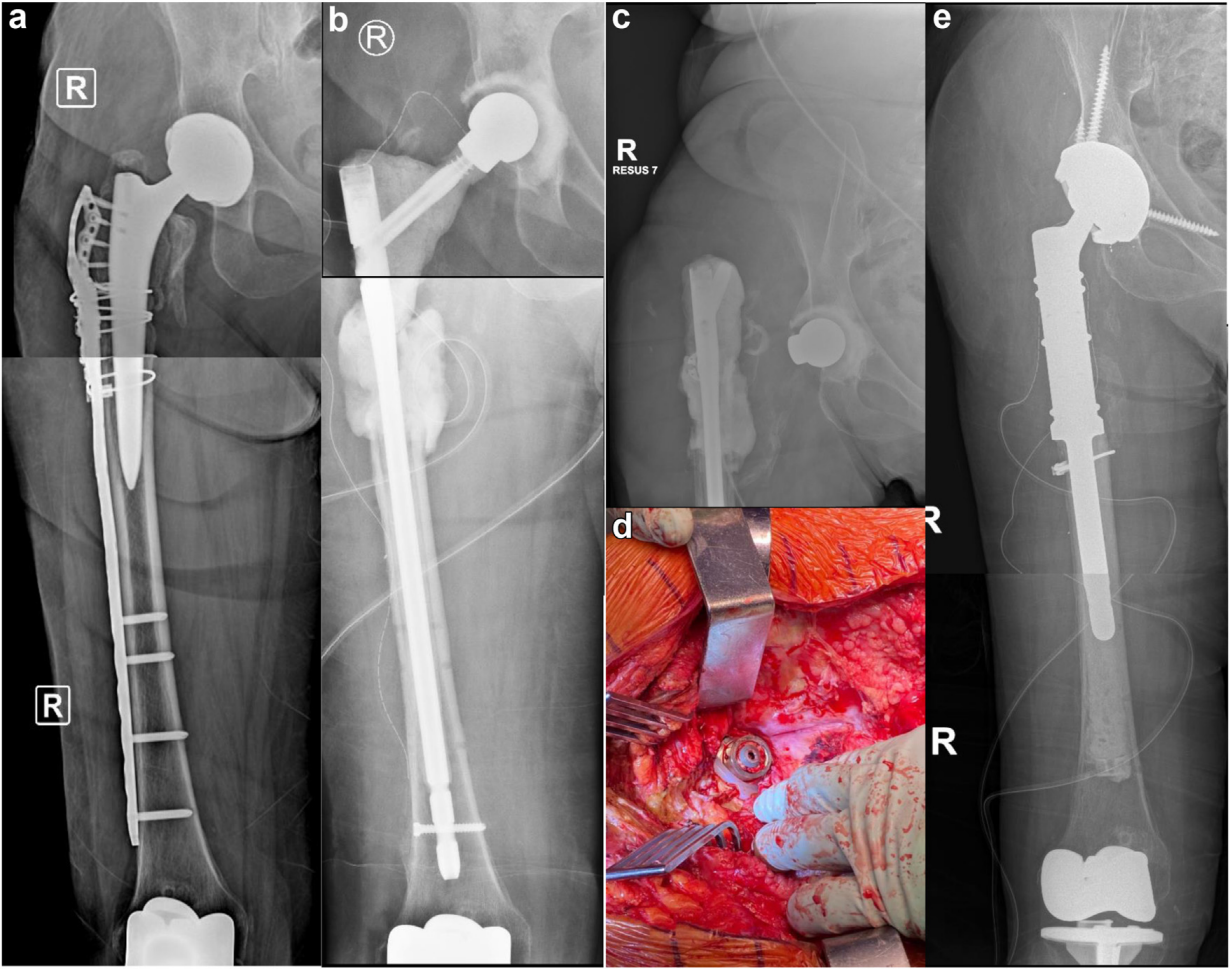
Case 3 RAH. (a) AP radiographs demonstrating infected right interprosthetic plate with nonunion of the lesser trochanter. (b) AP radiograph after the first-stage reconstruction of the femur using an antibiotic-coated-gamma nail as a temporary prosthesis. (c) AP radiograph demonstrating fractured implant prior to second-stage revision. (d) Intraoperative image shows the retained screw threads in the femoral head. Note the absence of metallosis. (e) AP radiograph of second-stage revision to proximal femur replacement prosthesis.
